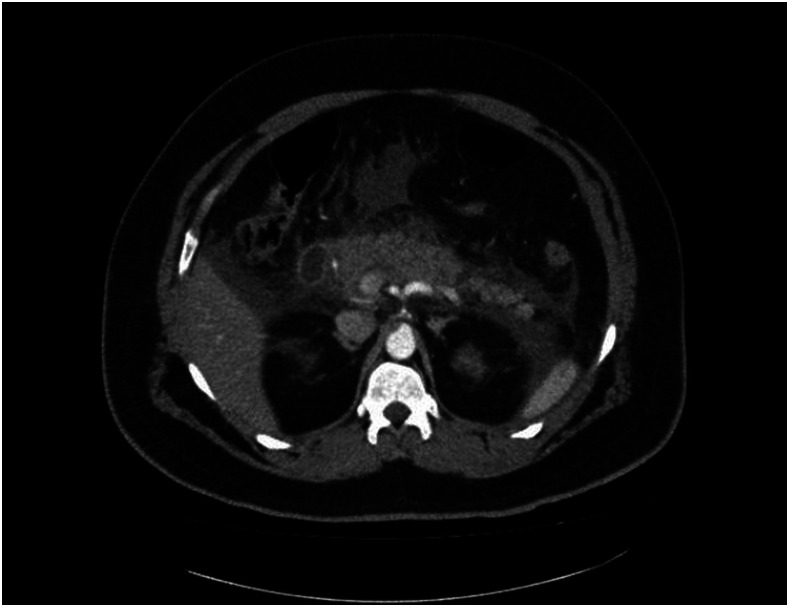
CT scan showing a swollen pancreas with inflammatory changes in surrounding areas.
